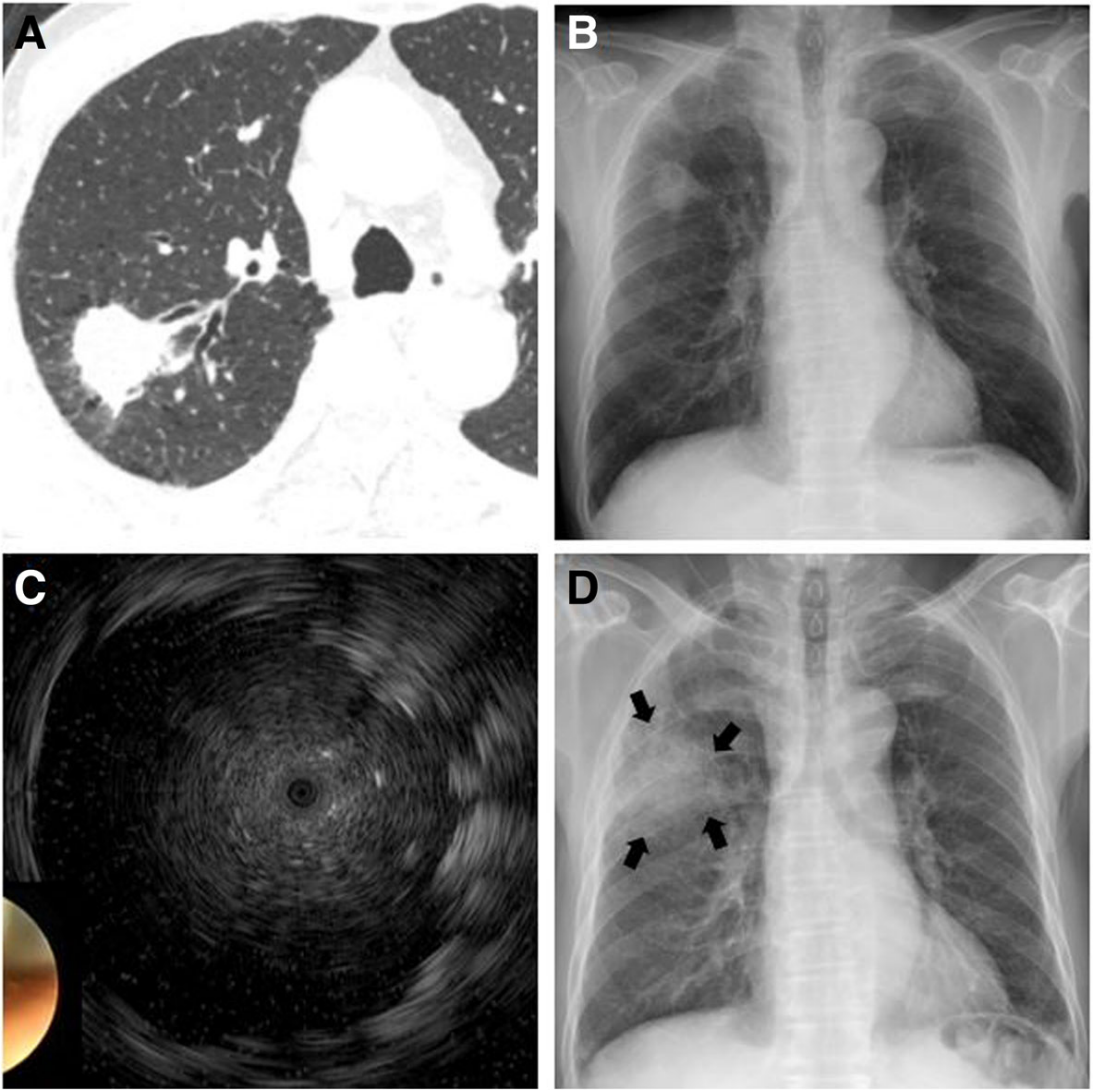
A patient who developed pneumonia after the procedure. a and b A patient was admitted with a nodule located in the right upper lobe and measuring 26.7 mm at its greatest diameter on a chest radiograph and computed tomography scan. c A radial probe EBUS placed within the target lesion showed a hypoechoic area with numerous hyperechoic dots. d Chest radiographs on day 5 showed an increased pneumonic consolidation (arrow) around the suspected tumor in the right upper lobe
